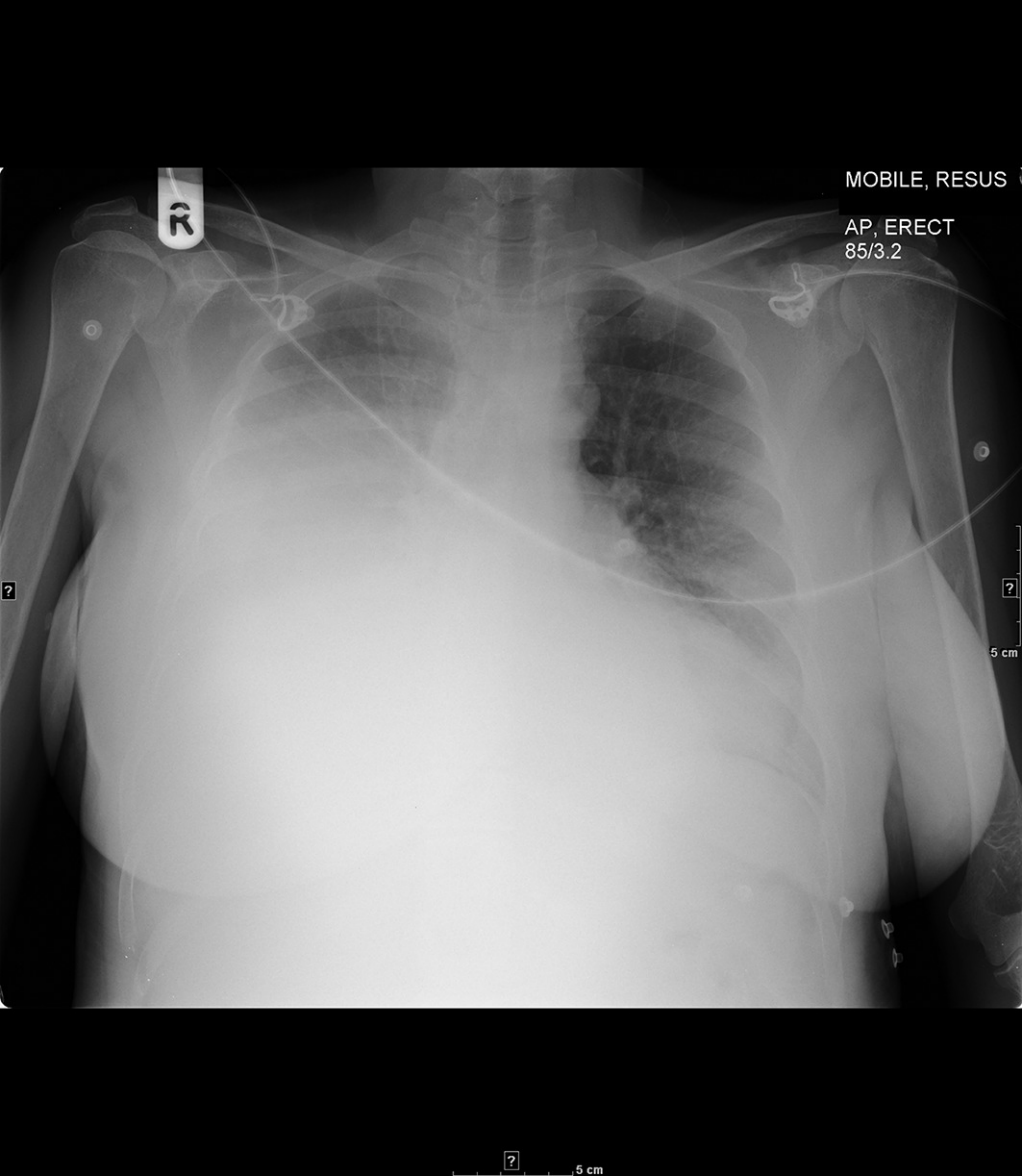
Chest X-ray at presentation shows a large right pleural effusion.
